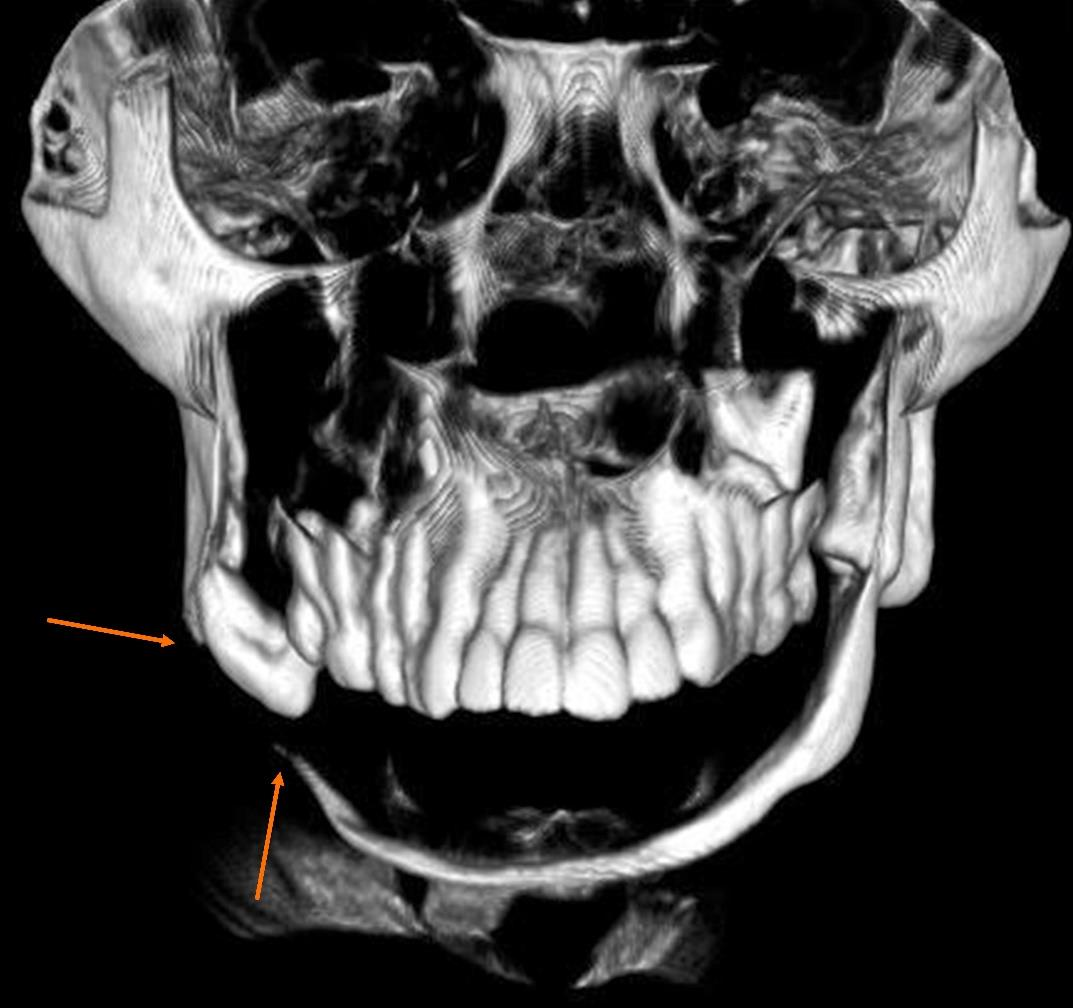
Coronal 3D-CBCT image showing resorbed mandible and pathological fracture of right lower border of mandible (orange arrows).
